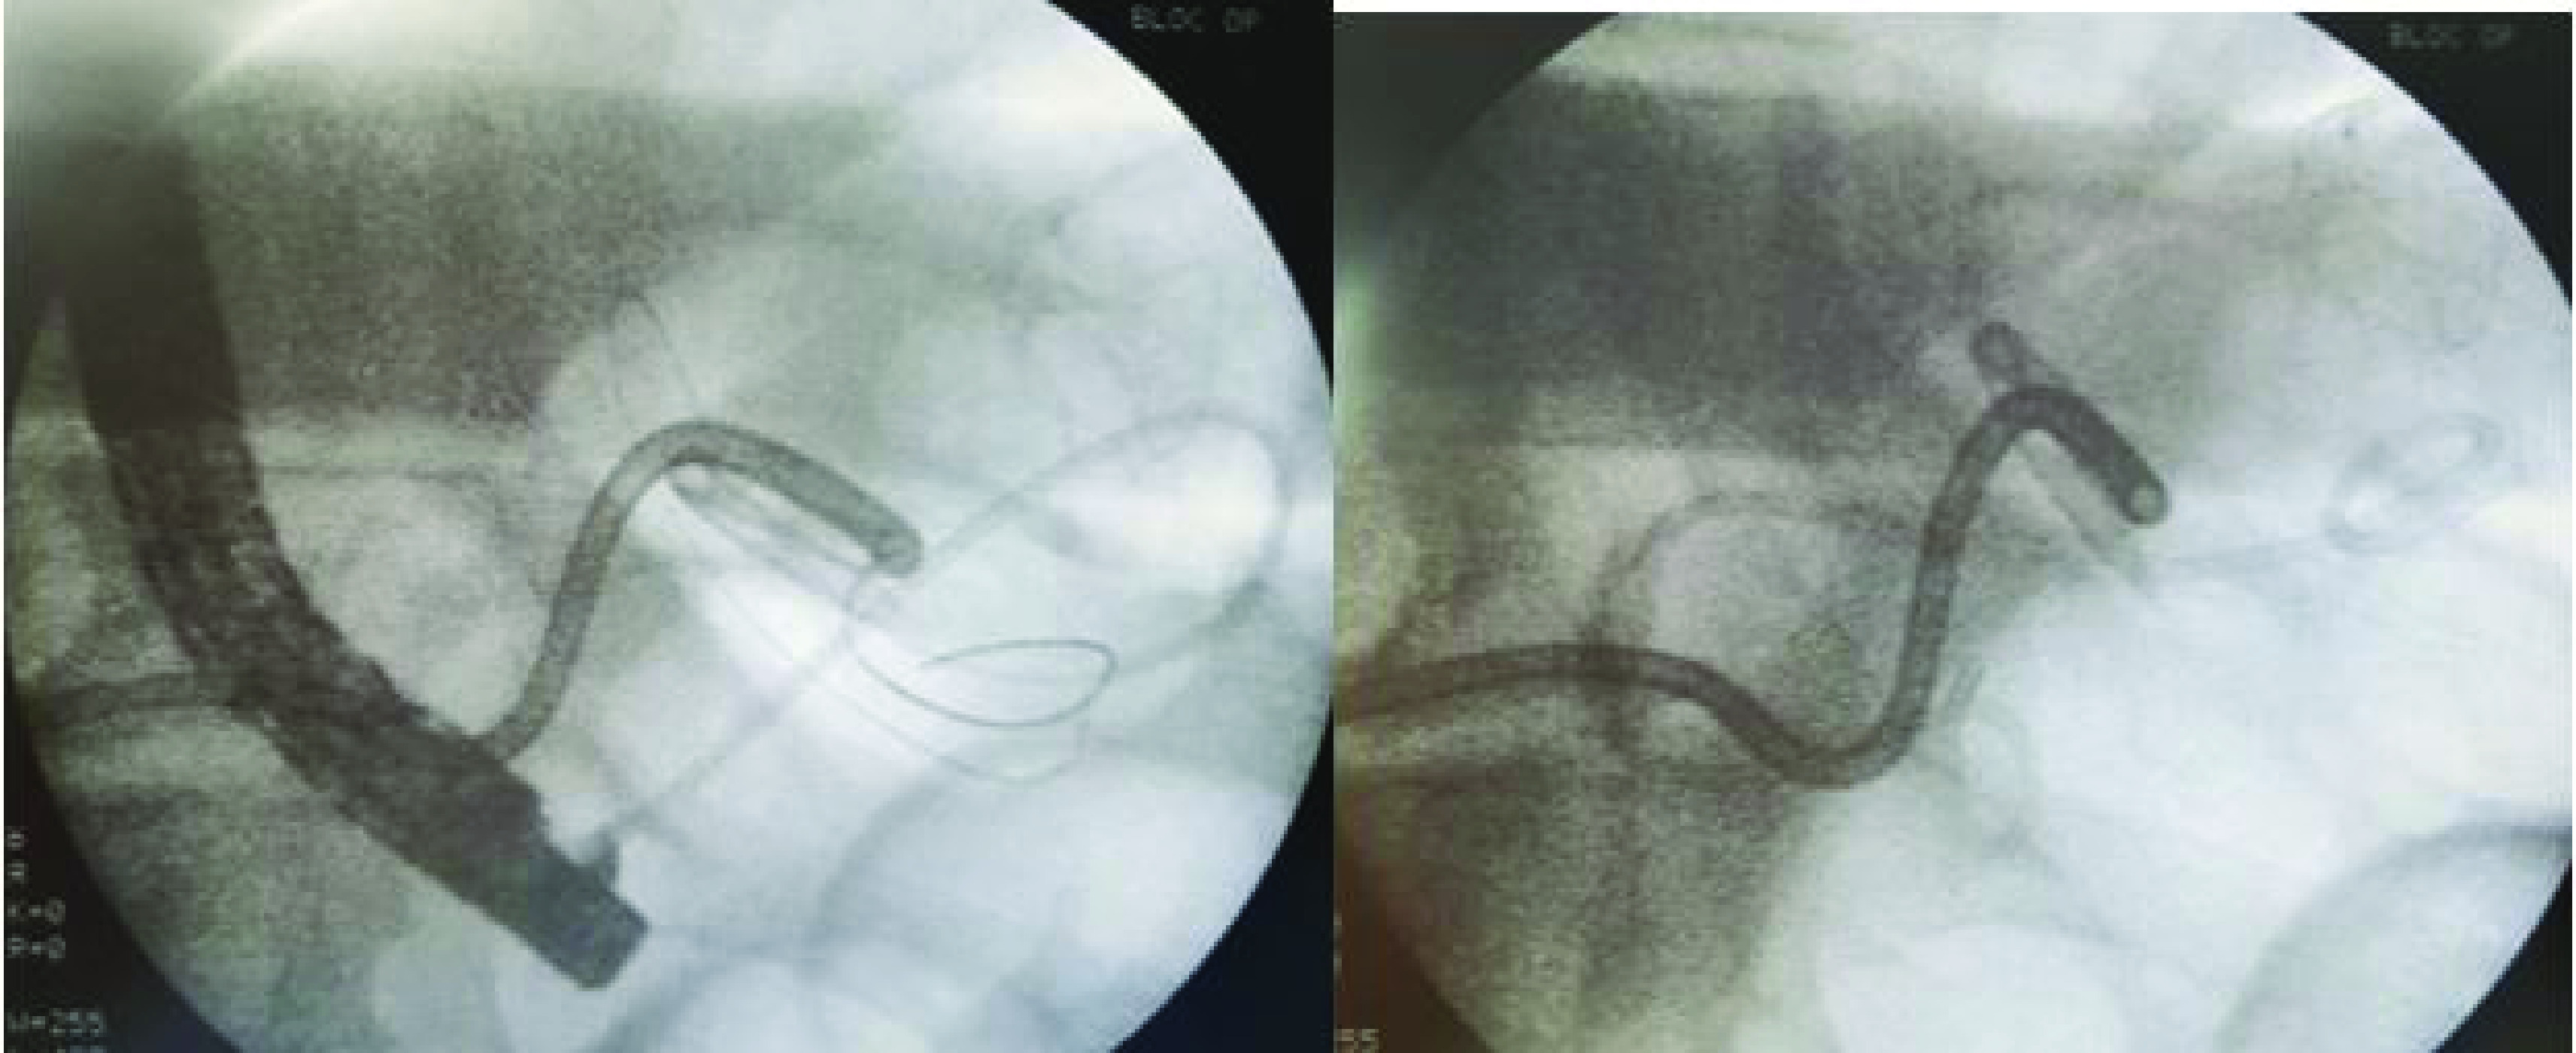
X-ray showing the endoscopic internal drainage with double pigtail plastic stent.
